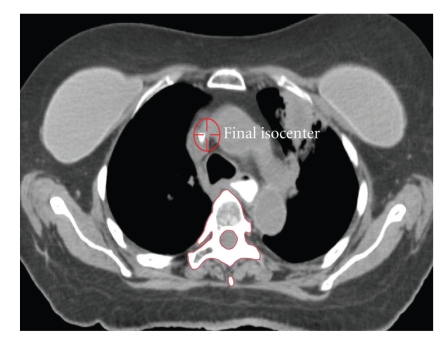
Volumetric on-board Kilovoltage cone-beam CT imaging.
